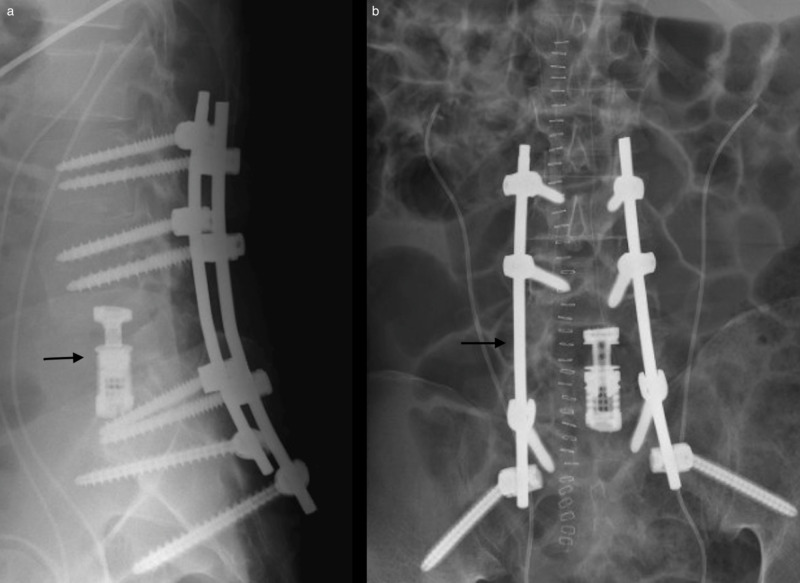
X-rays of postoperative hardware (a) Lateral x-ray demonstrating fused interbody cage between L4 and S1. (b) Posteroanterior x-rays demonstrating postoperative hardware with rods, bilateral sacroiliac screws, and pedicle screws extending from L3 to S1.
